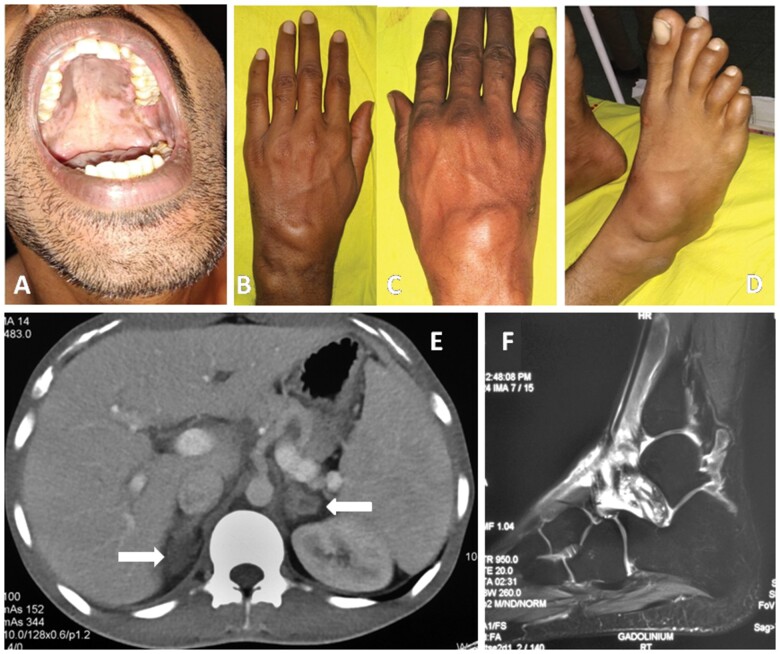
Clinical presentation and diagnostic evaluations. (A) Brownish black pigmentation of the palate. (B) Tenosynovitis of extensor tendons over left wrist joint. (C) Tenosynovitis of extensor tendons over right wrist joint. (D) Tenosynovitis of flexor tendons over right ankle joint. (E) CT showing bilateral enlarged and hypodense adrenals. (F) MRI post contrast images of the ankles showing mild joint effusion with enhancing synovial thickening with tenosynovitis of extensor digitorum tendons
